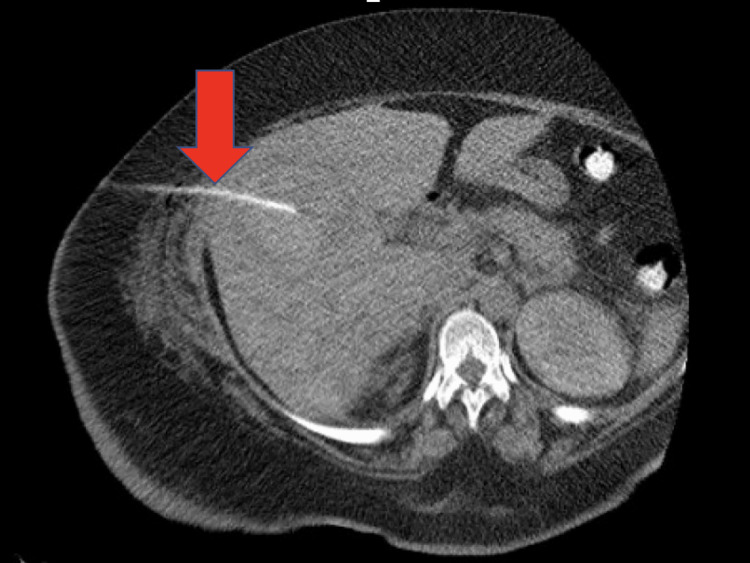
CT-guided drainage of hepatic collection. The red arrow shows the needle. CT: computed tomography
